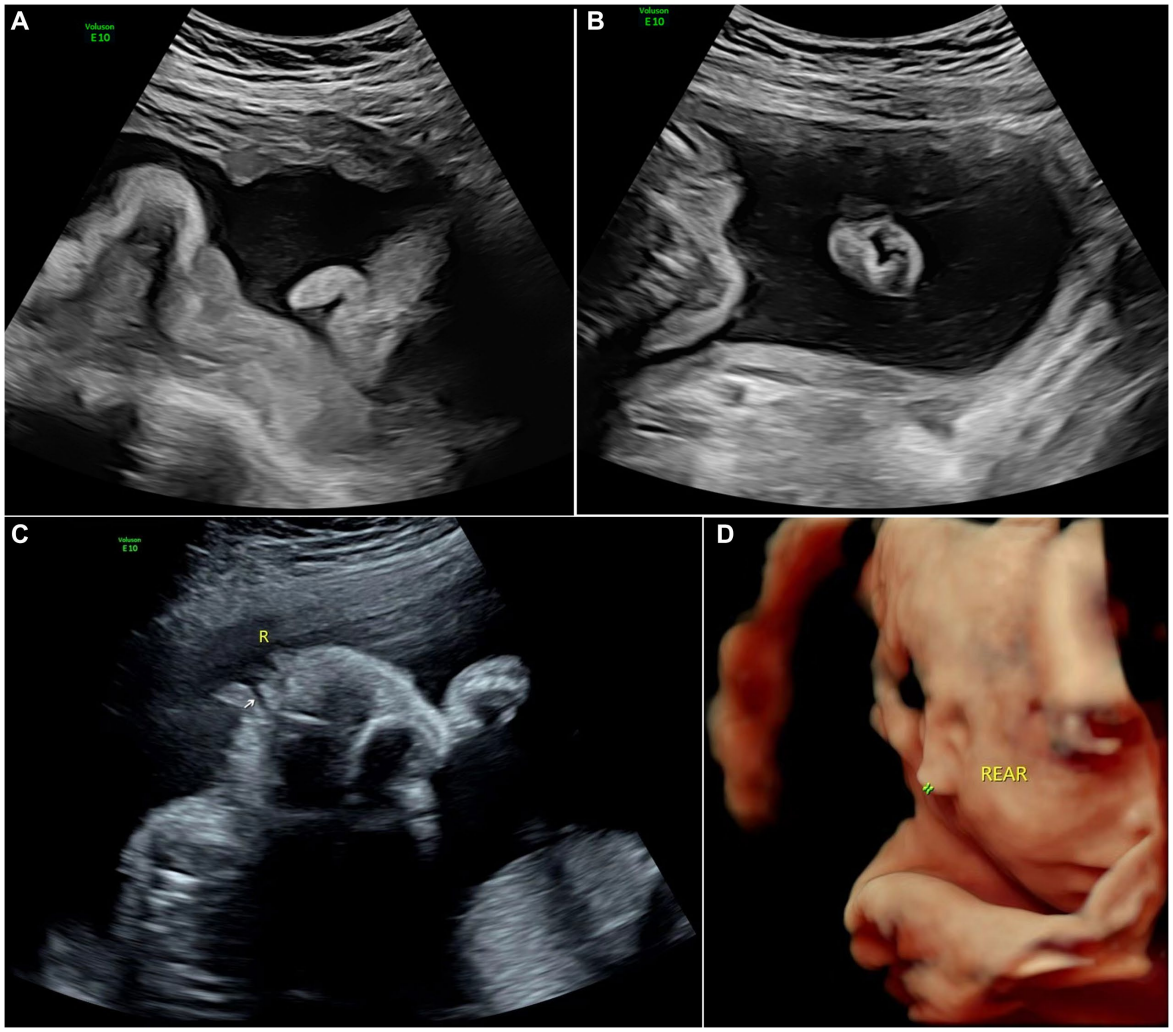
Images of type II of microtia: (A) 2D sonography of microtia; (B) 2D sonography of contralateral ear(normal); (C) 2D sonography of unilateral microtia type II with normal external acoustic meatus; and (D) 3D sonography of Unilateral microtia type II.
